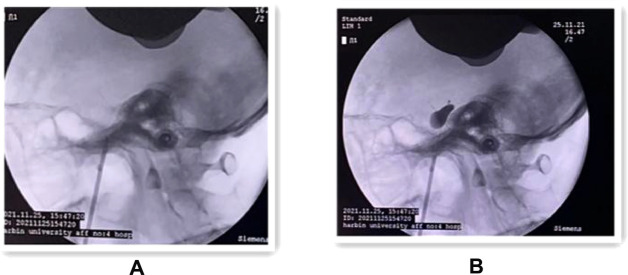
(A) Intraoperative lateral x-ray fluoroscopic view showing the successful puncture of the trocar into the internal orifice of the foramen ovale. (B) Lateral x-ray fluoroscopy showing the “pear-shaped” balloon in the filled state.
